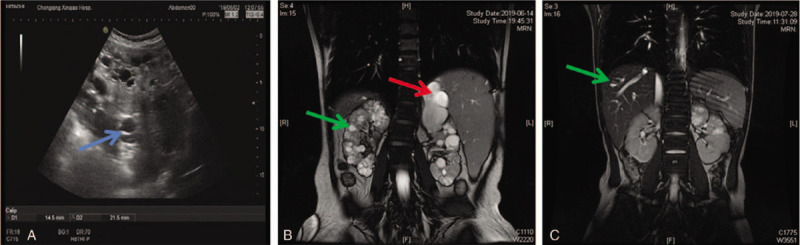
Clinical diagnostic images of proband. A: Abdominal ultrasound imaging showing the renal cysts of the proband (arrow). B: Magnetic resonance imaging showing renal cysts (green arrow), liver cysts and intrahepatic bile duct dilation of the proband (red arrow). C: Magnetic resonance imaging showing intrahepatic bile duct dilation of the affected sib (green arrow).
